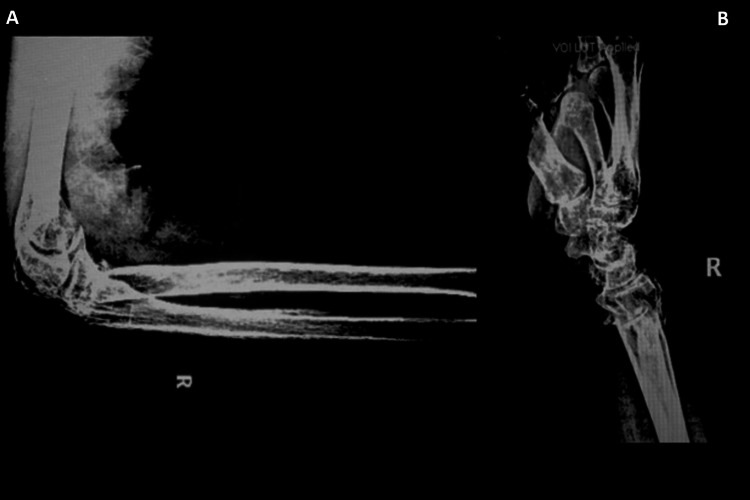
Anteroposterior (A) and lateral (B) elbow and proximal forearm radiography done 12 weeks postoperatively. Radiographs showing good healing and alignment maintained.
